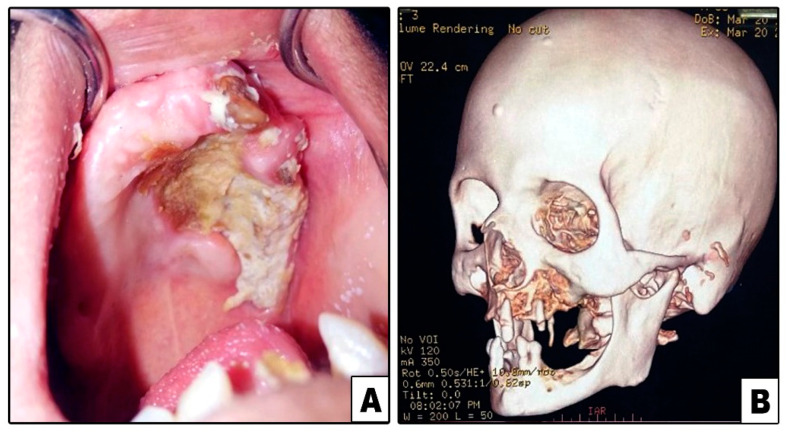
Case#7: (A) Exposed necrotic palatal and alveolar bone on left side. (B) 3D CT showing bone destruction involving left maxilla and lower part of zygoma.
